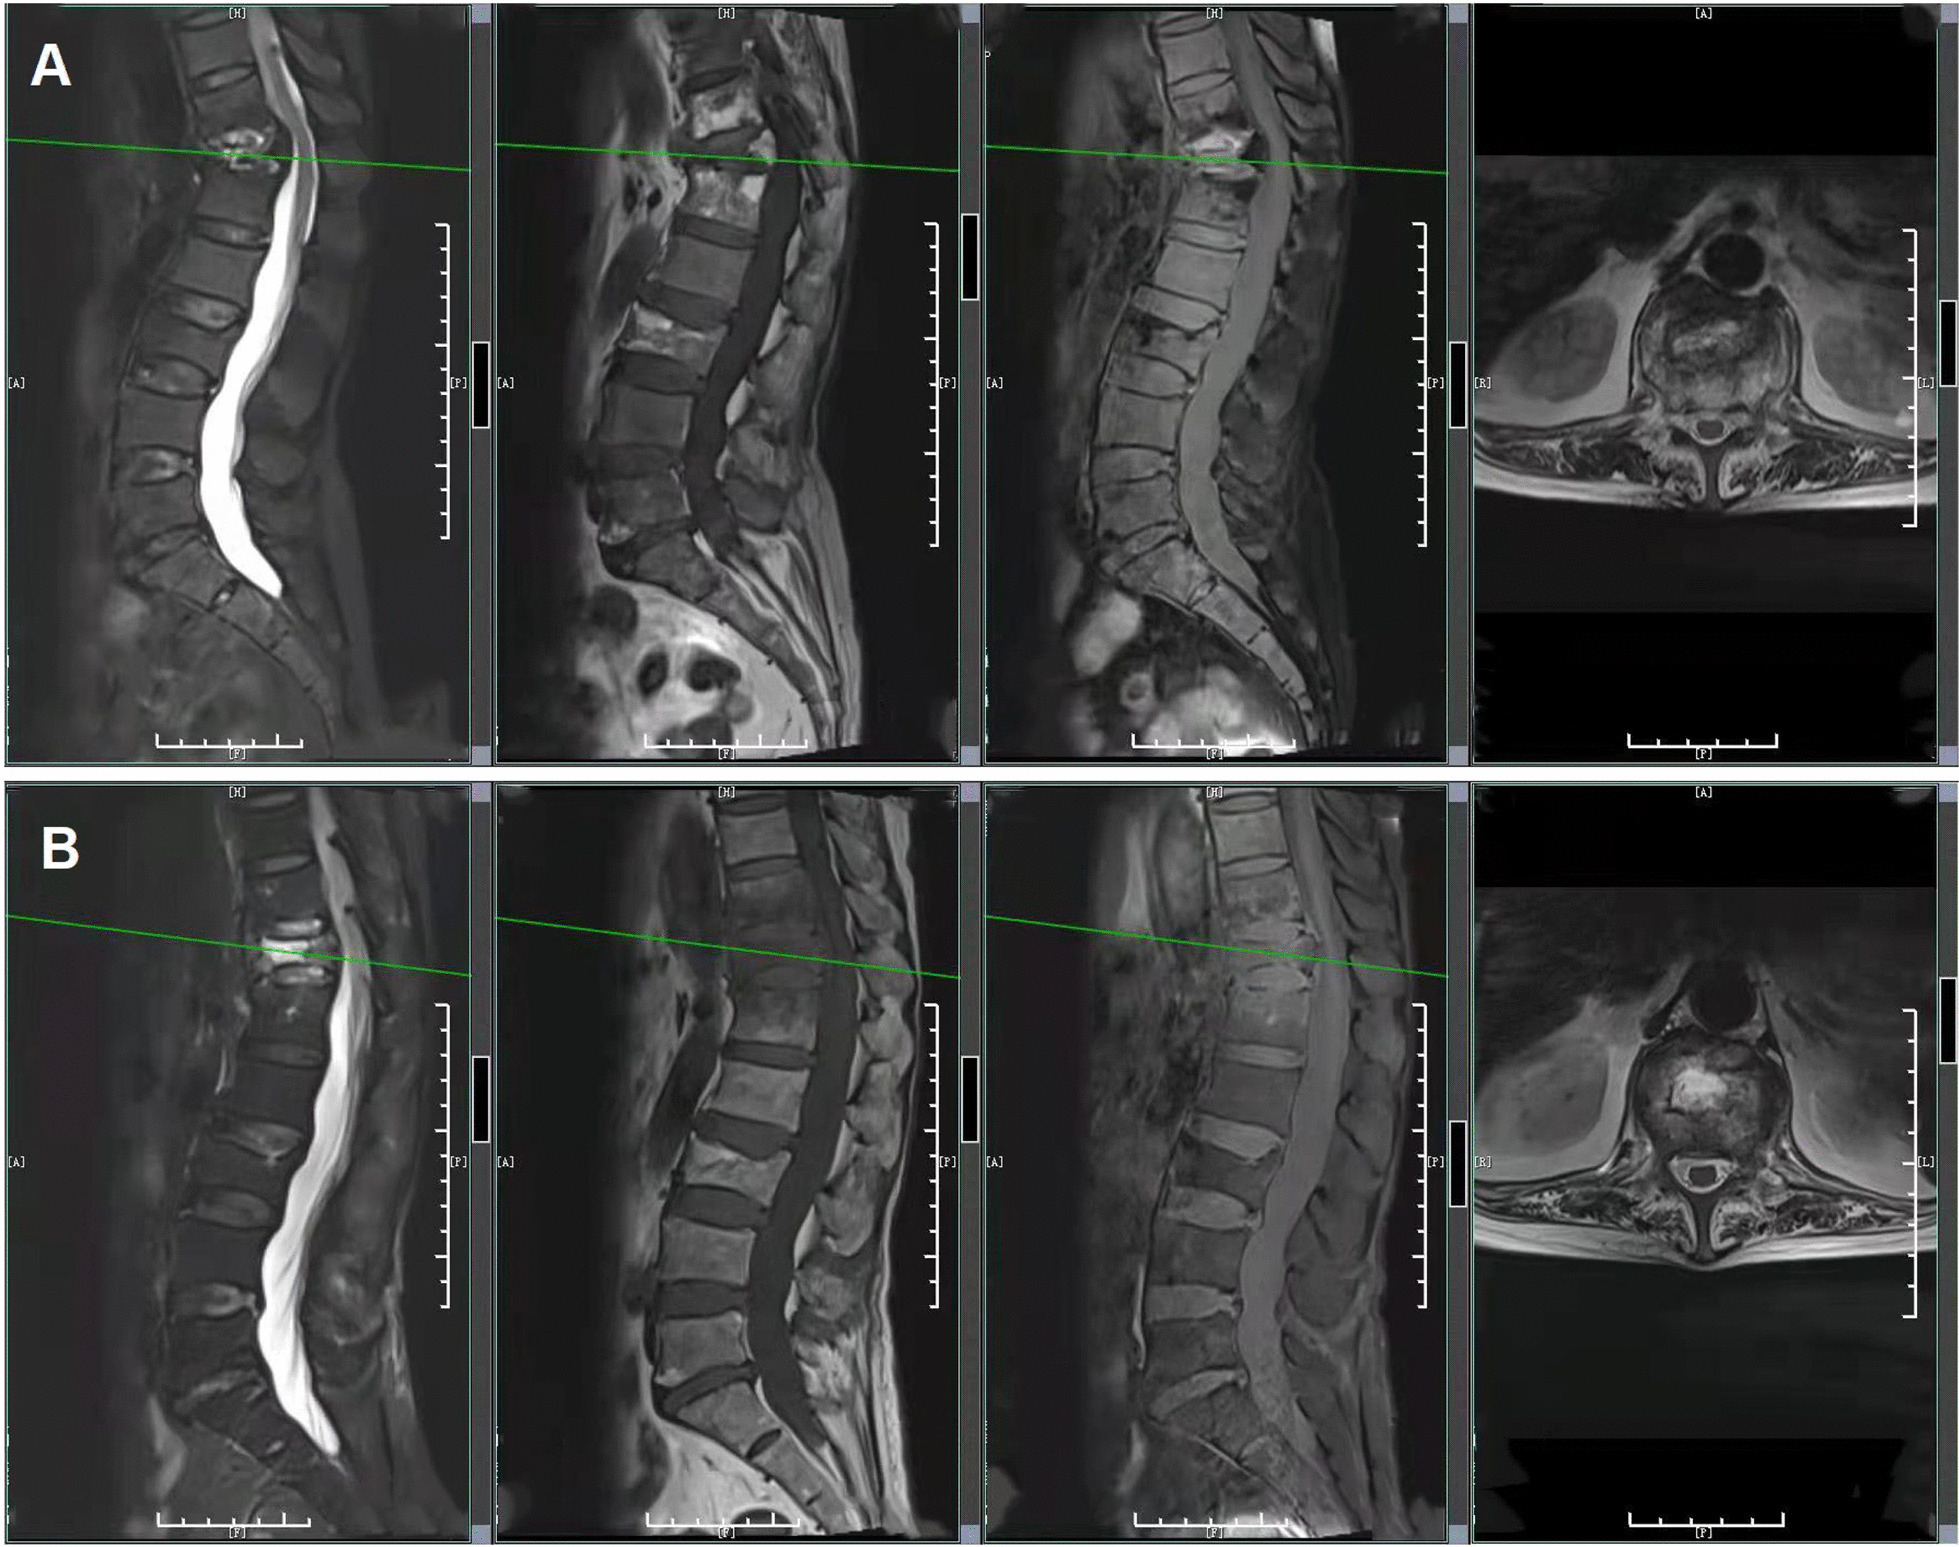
Results of the MRI in lumbar and thoracic vertebrae. A MRI of the lumbar and thoracic vertebrae on September 17, 2020. B MRI of the lumbar and thoracic vertebrae on October 10, 2020
